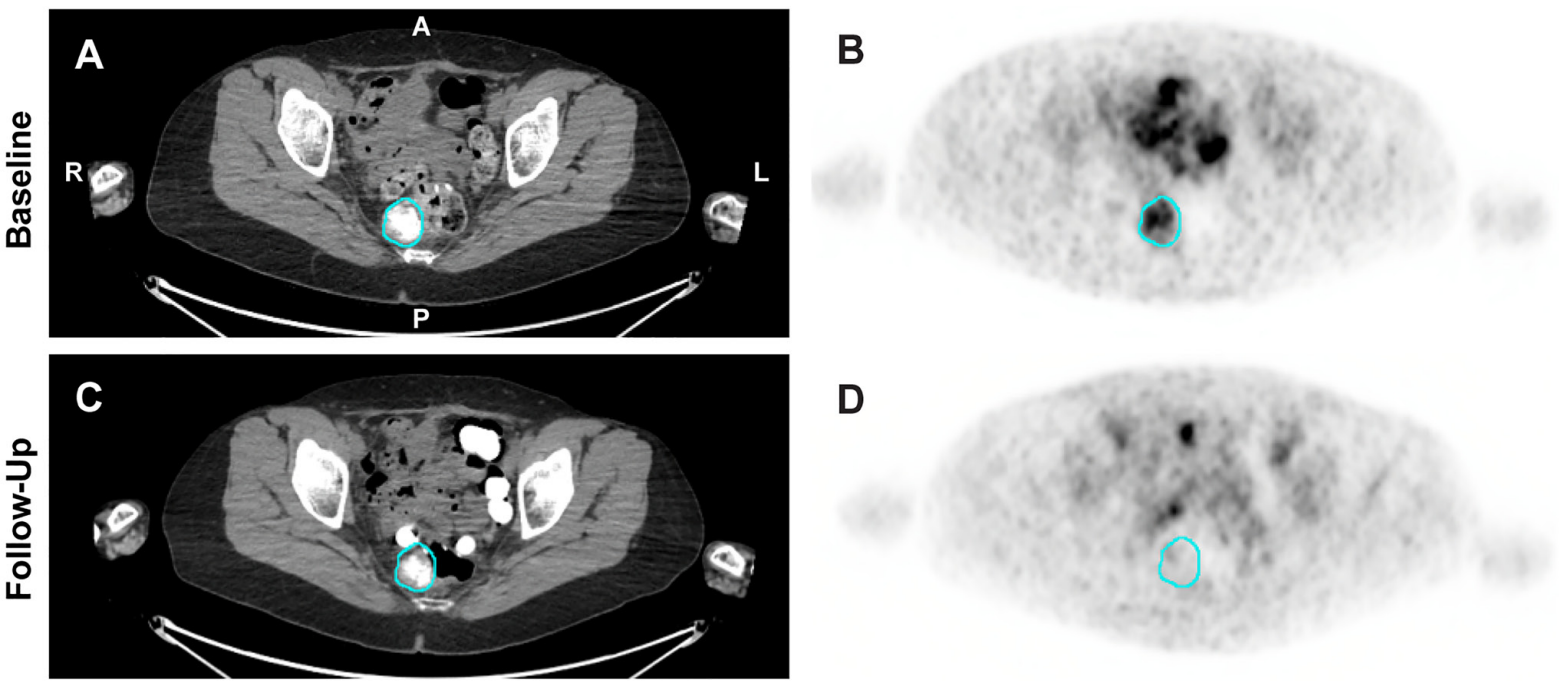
^18^F-FES PET/CT images from patient 40 with serous ovarian cancer. Imaging was performed prior to treatment ((A) [CT] and (B) [PET]) and after 3 days of treatment with Z-endoxifen at DL8 ((C) [CT] and (D) [PET]). The blue circles indicate the location of a pre-sacral soft tissue lesion.
